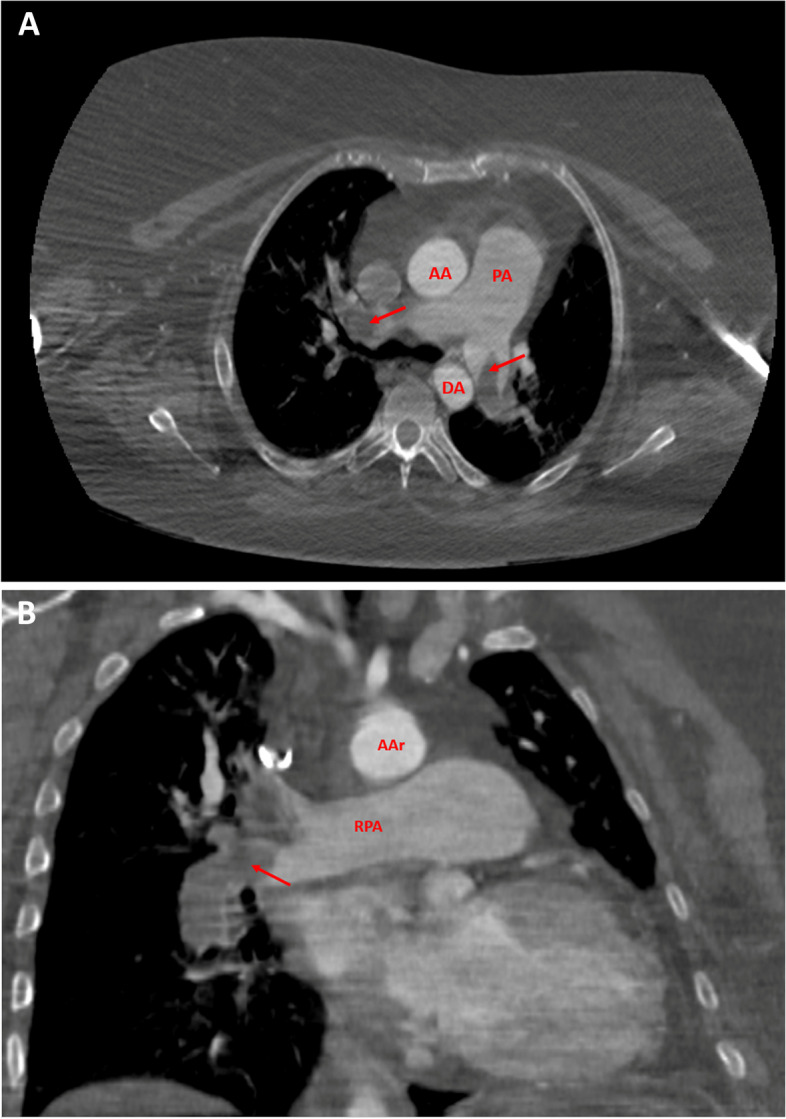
A-B Computed tomography pulmonary angiogram in axial scan (A) coronal scan (B). Bilateral pulmonary artery emboli are shown by red arrows. AA = ascending aorta. AAr = aortic arc.; DA = descending aorta. PA = pulmonary artery. RPA = right pulmonary artery
